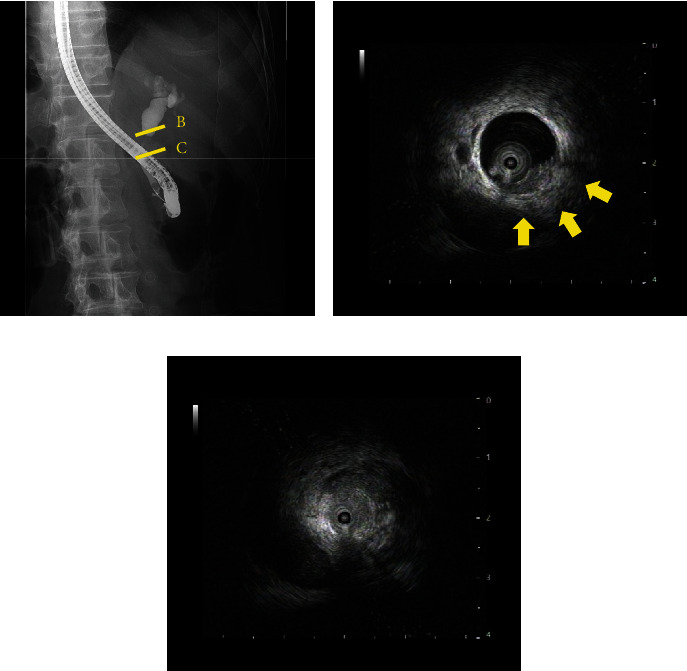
ERCP and IDUS findings. (a) ERCP showed a filling defect of the distal bile duct; the cystic duct was invisible. (b) IDUS showed that the tumor had invaded the bile duct subserosa (yellow arrow). (c) The patient's distal bile duct was almost obstructed by the tumor. ERCP: endoscopic retrograde cholangiopancreatography; IDUS: intraductal ultrasonography.
